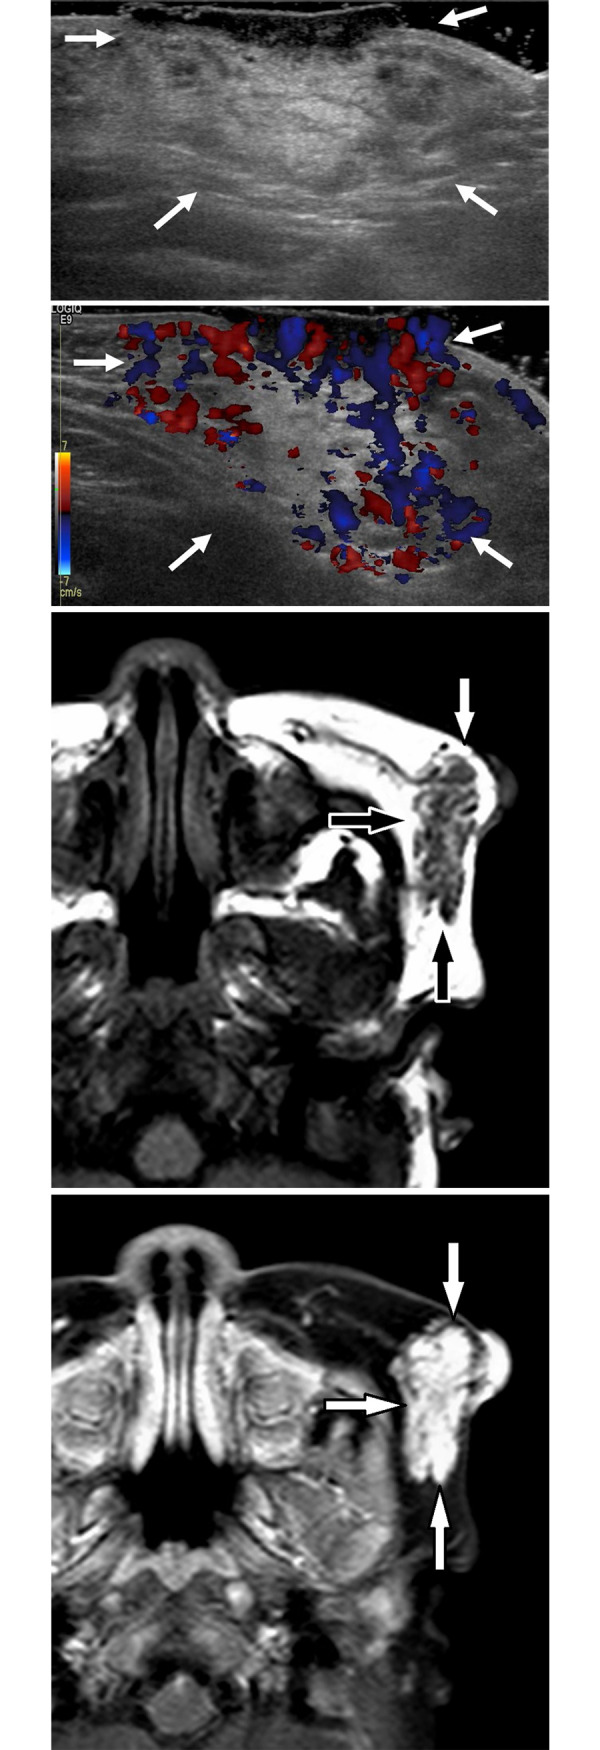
Image from a 4-month-old girl with infantile hemangioma who showed incomplete regression after 1 year of propranolol treatment. (a) Gray-scale US image shows a hyperechoic subcutaneous mass with a thick skin lesion (arrows). (b) Color Doppler image reveals hypo vascularity within the lesion (arrows). (c) Axial T1-weighted MRI of the face shows a lobulated mass (arrows) with multiple fatty septa. (d) Axial T1-weighted fat-suppressed contrast-enhanced MR image of the face reveals homogeneously strong enhancement (arrows).
